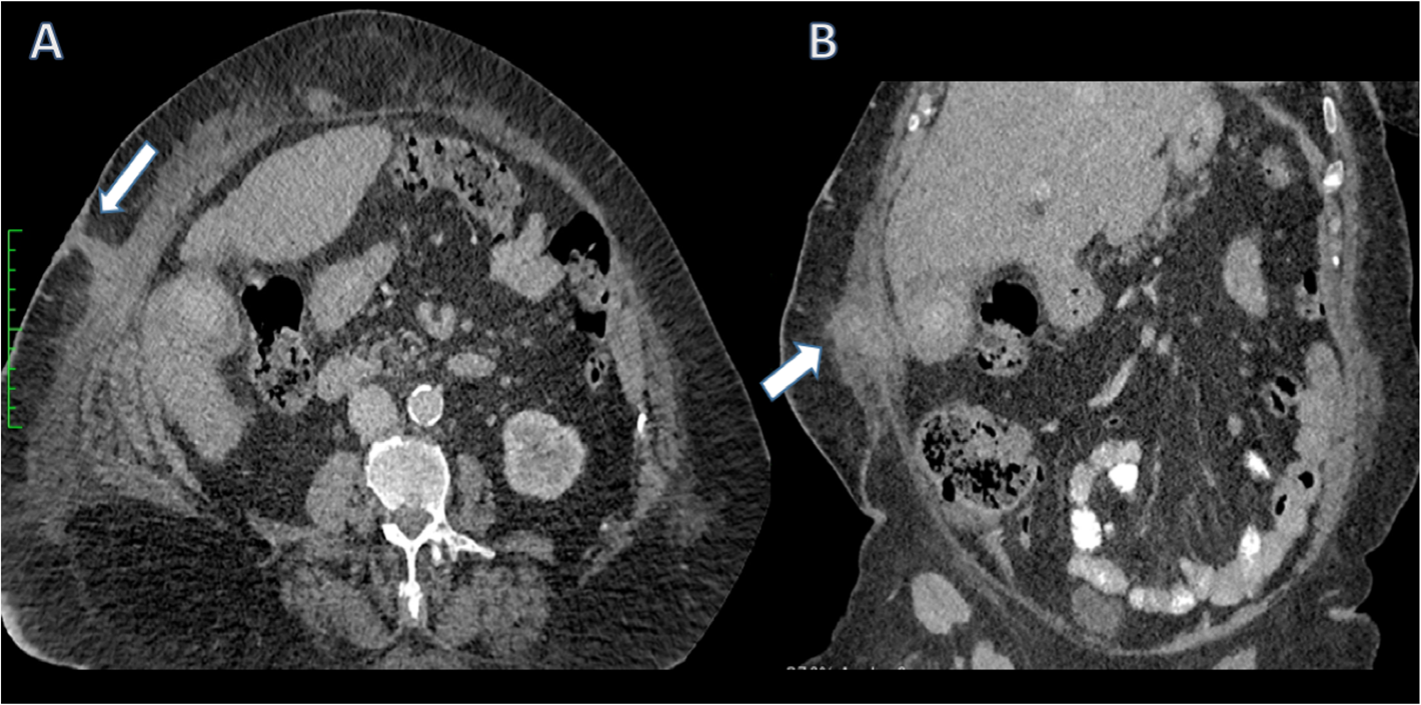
Axial (a) and coronal (b) contrast-enhanced CT of the abdomen. There is extensive gallbladder wall thickening and stranding with the inflammatory process extending through the peritoneum to the right anterolateral abdominal wall with fistulation to the skin (arrows)
